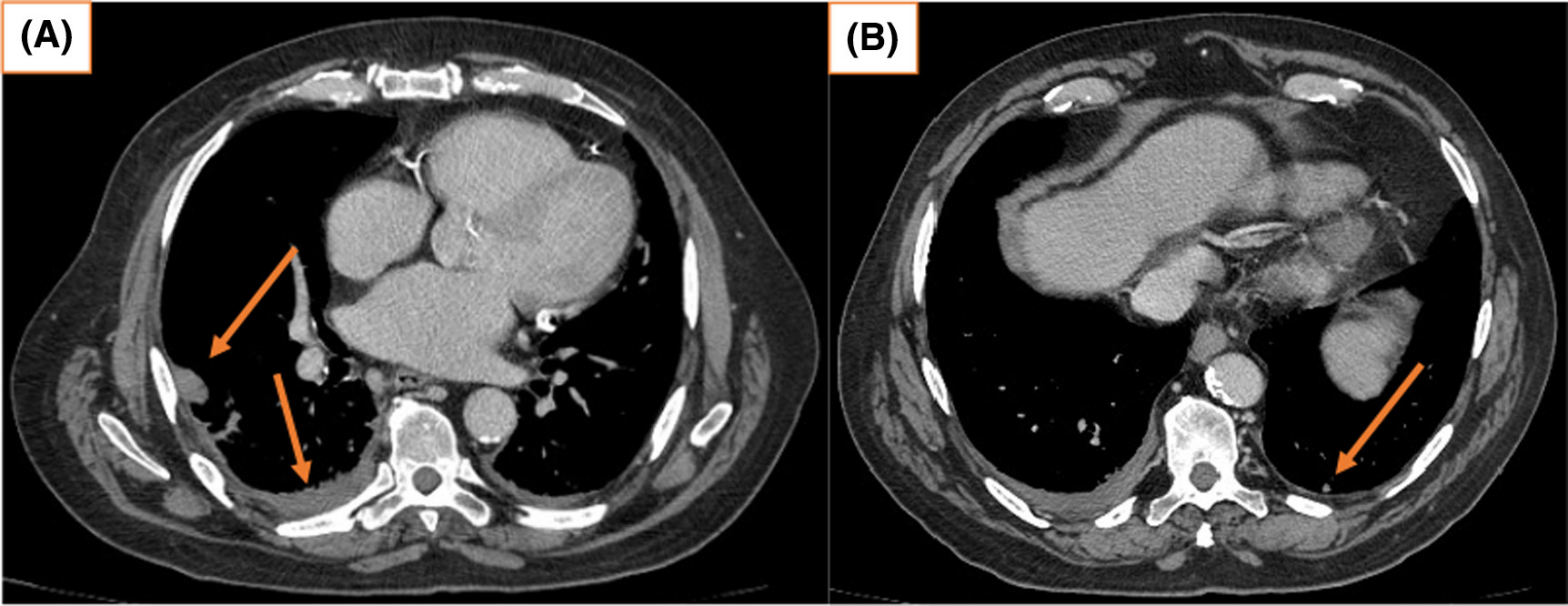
(A, B) January 2023, chest CT 6 months into systemic immunotherapy demonstrating ongoing partial response.
